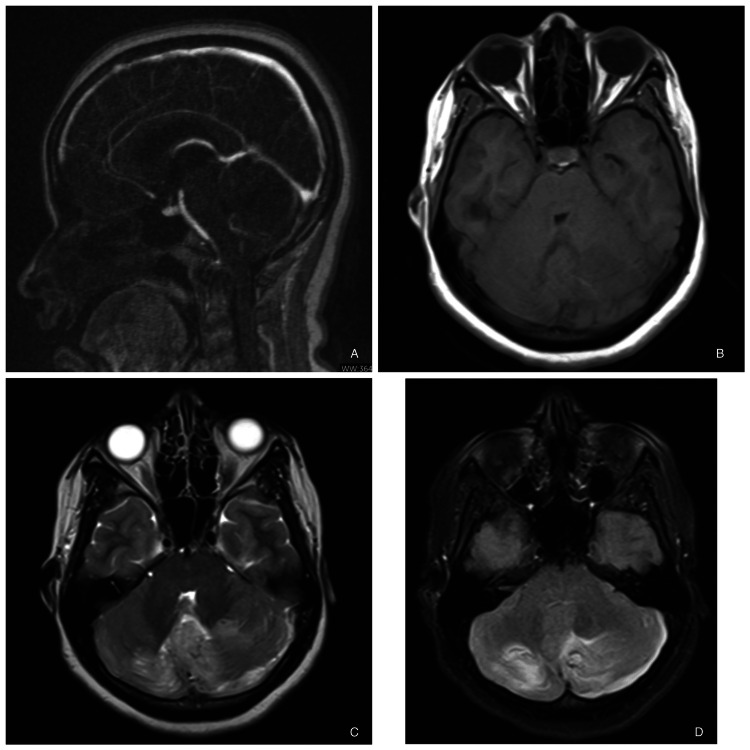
(A) MR venogram showing no signs of cerebral venous thrombosis, (B) MRI brain T1W image showing hyper-intensity in bilateral cerebellum, (C) MRI brain T2W image showing hyper-intensity in the same area, (D) MRI brain FLAIR showing hyperintensity in the same region as mentioned in T1. FLAIR - Fluid-attenuated inversion recovery
